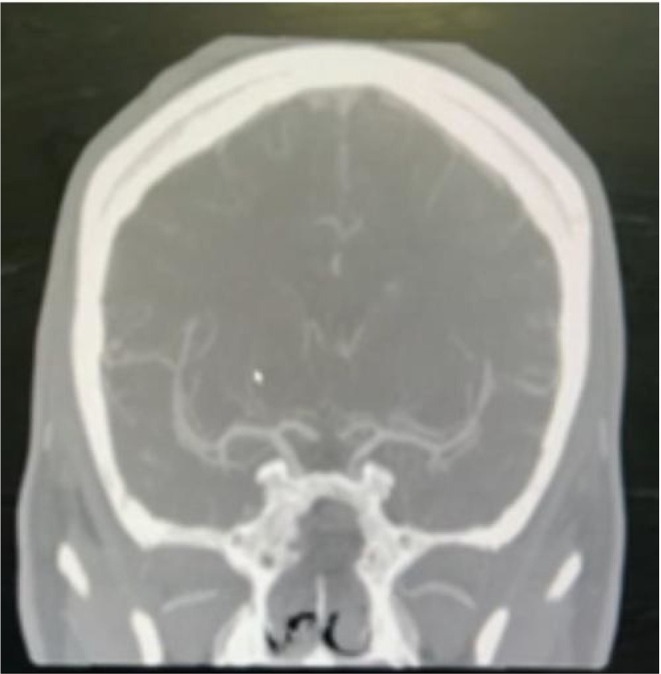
Patient's brain CT angiography that showed a patent stent with no signs of new aneurysm formation.
